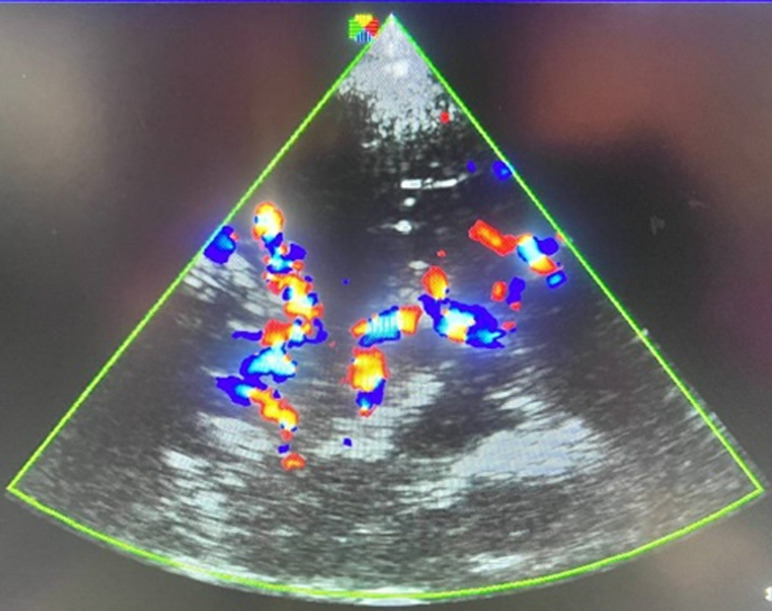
structure of the circle of Willis on color Doppler ultrasound
